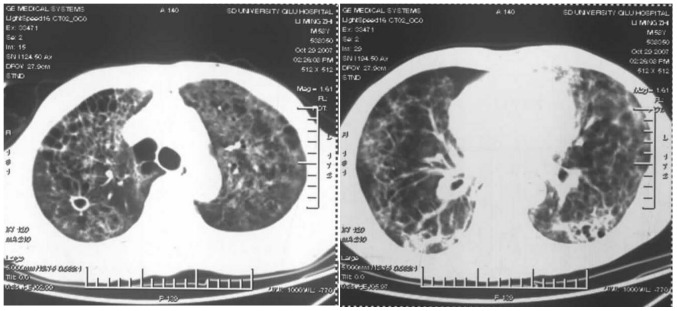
Chest computed tomography on October 29, 2007: Diffuse reticular patchy shadows; nodule shadows have become large (evident in the central region) and lobe cyst-like shadows are present in the right upper lungs.
